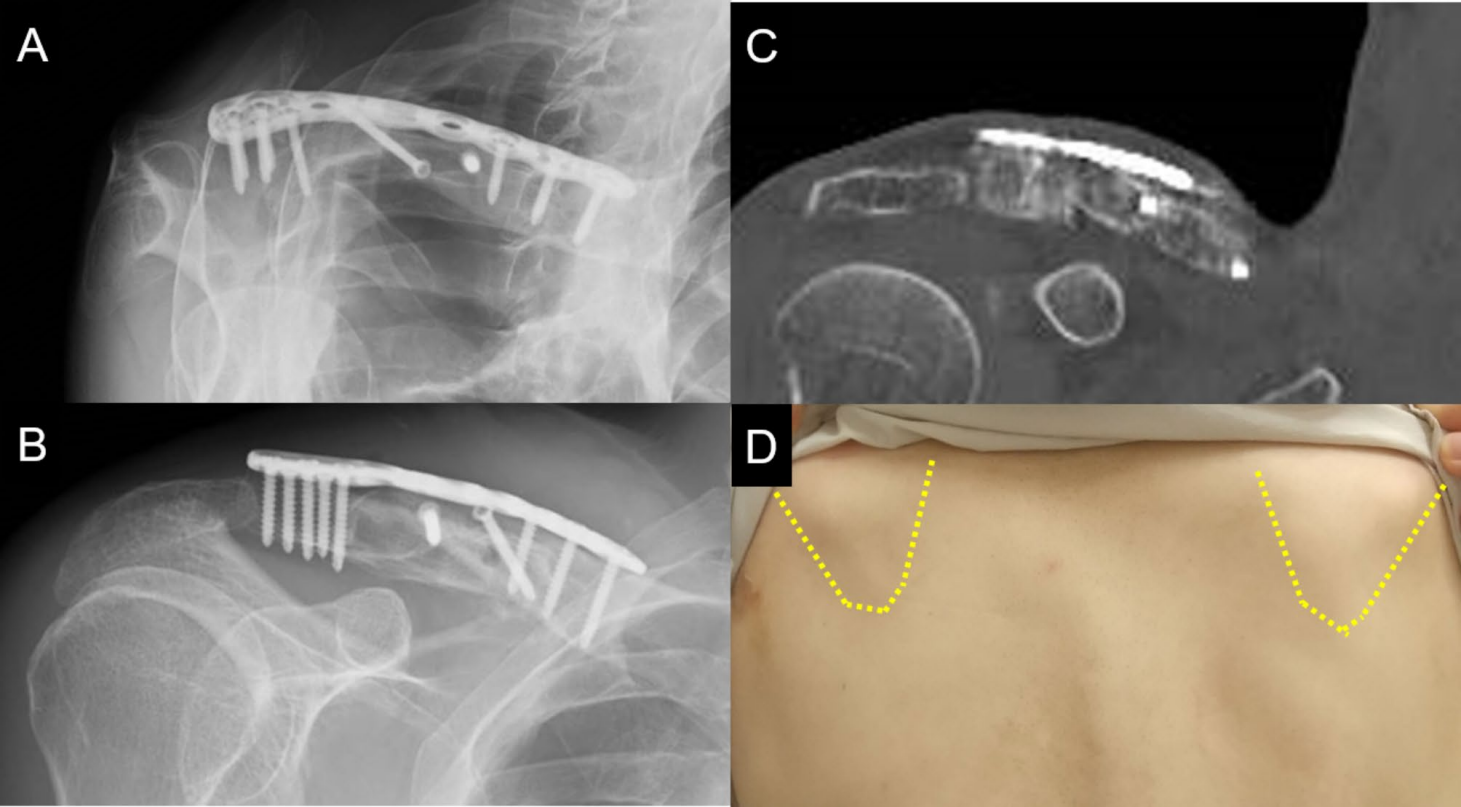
Postoperative imaging and physical findings. Radiographs of the right clavicle after plate fixation with parallelogram prism bone grafts (A and B). Computed tomography at 9 months after surgery showing bone union (C). Appearance of scapular showed that the medial and inferior translocation of superior angle of the scapula and protrusion of the inferior scapular angle subsided (D)
